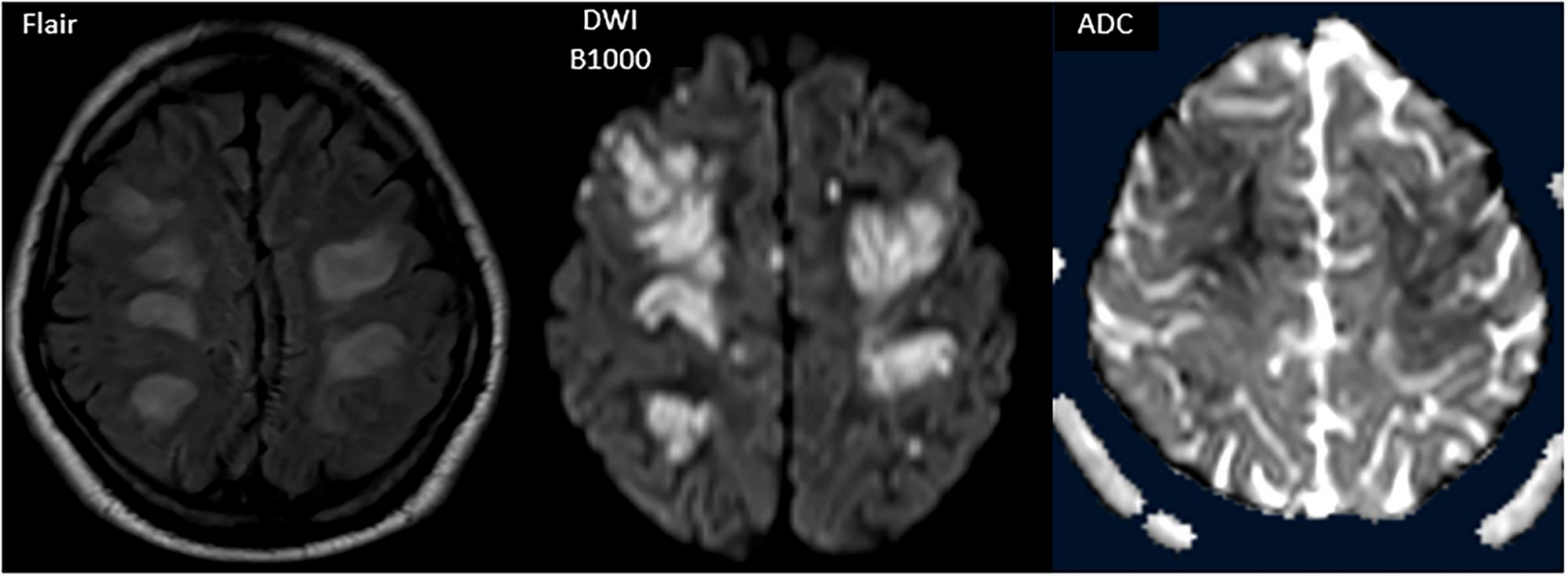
T2-weighted FLAIR and diffusion-weighted imaging (b = 1000 s/mm^2^) with apparent diffusion coefficient (ADC) mapping, passing through the convexity white matter. Signal abnormalities are seen in the white matter as FLAIR hyperintensity with corresponding diffusion restriction, which is notably limited to the periphery of the larger lesions as confirmed on the ADC map.
